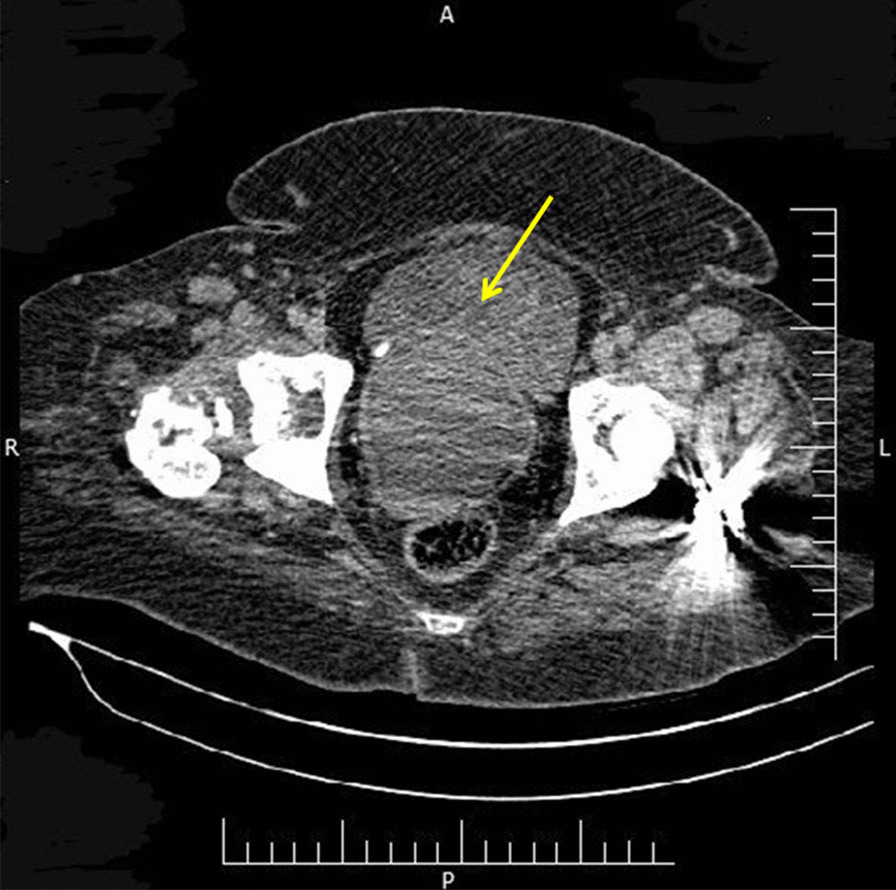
A large mass (yellow arrow) in posterior wall of uterus in axial-view CT scan
